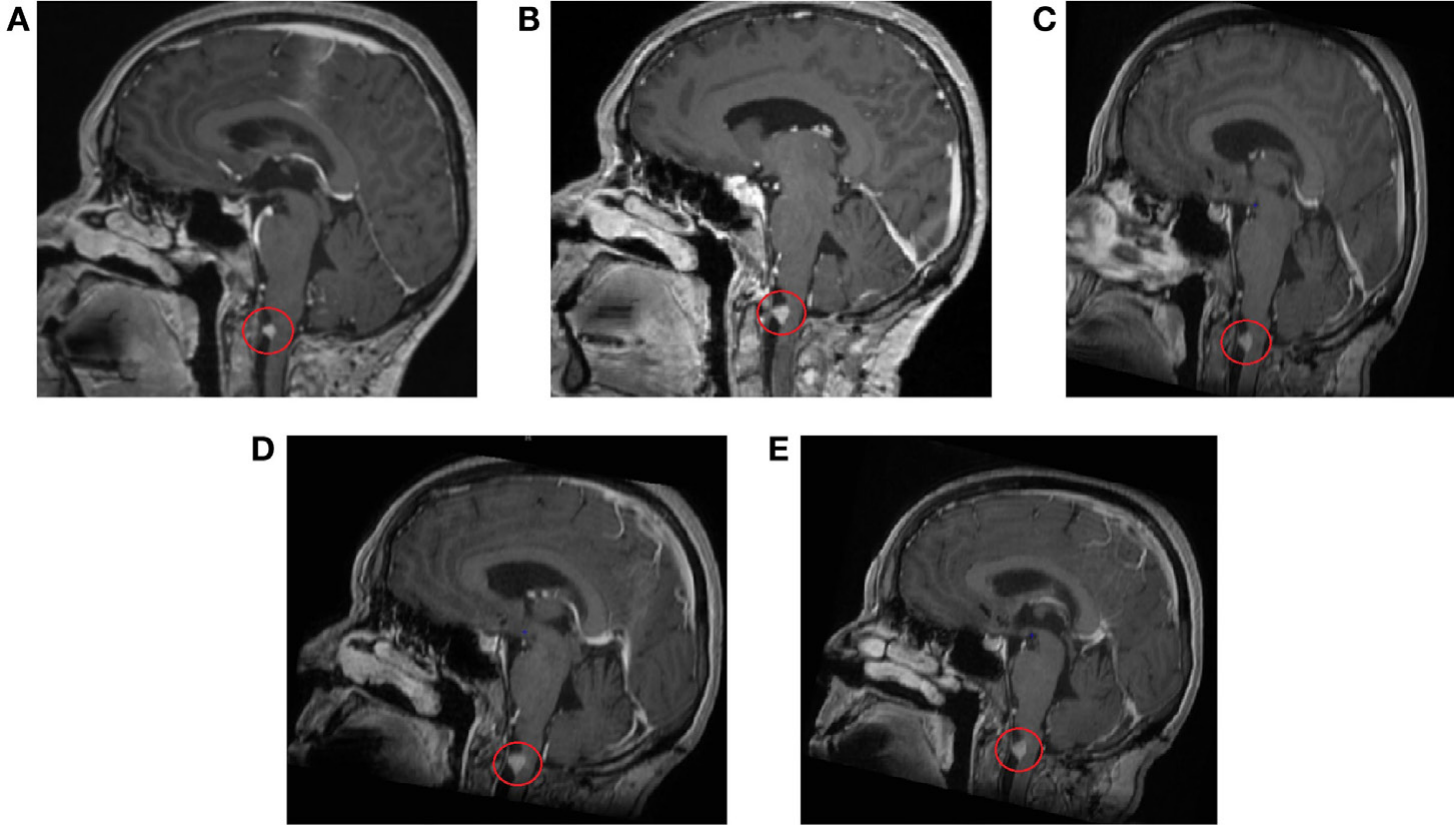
Contrast-enhanced magnetic resonance imaging scans of the head and brain show residual tissue of the melanoma metastasis without significant changes in structure and size. (A) 11 September 2014, (B) 5 March 2015, (C) 29 September 2015, (D) 29 February 2016, (E) 2 August 2016.
